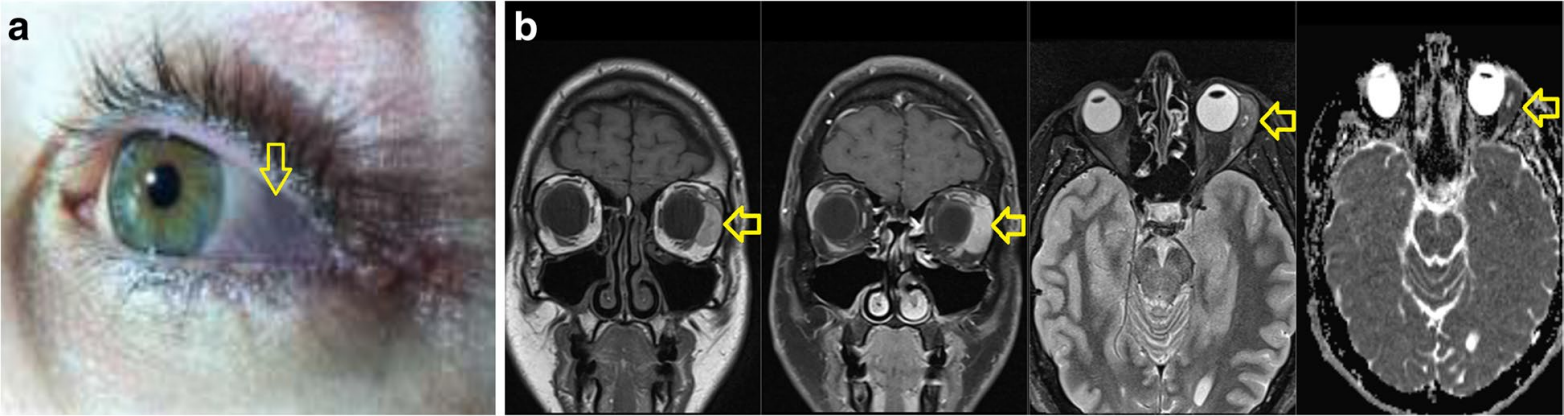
a Clinical photo showing an area of blue/grey discoloration and swelling (yellow arrow) involving the temporal bulbar conjunctiva, b MRI images from left to right - T1 coronal, T1 coronal with fat saturation and intravenous Gadolinium contrast, T2 Short Tau Inversion Recovery (STIR) axial and Diffusion Apparent Diffusion Coefficient (ADC) highlighting an intrinsically generally T1 hyper-intense, T2 hypo-intense contrast enhancing lateral retro-septal mass (yellow arrows) which exhibits restriction of water movement on diffusion imaging. This is positioned inferior to the lacrimal gland and lateral to the left globe which itself is mildly distorted by the lesion
